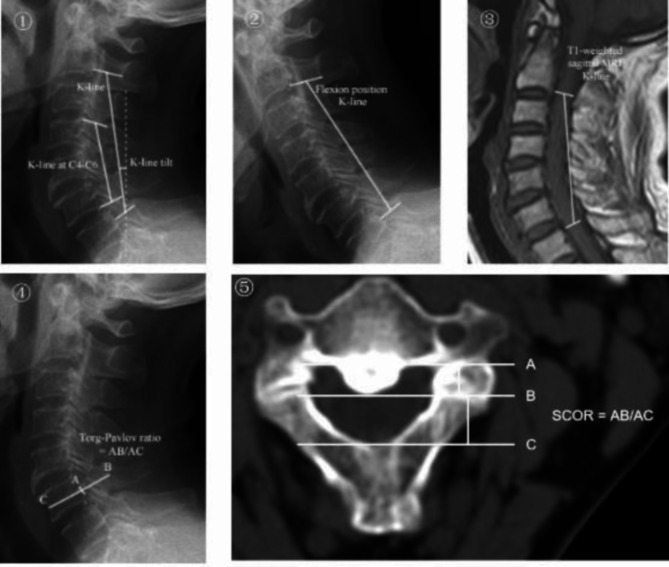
①. K-line is a straight line connecting the midpoints of the spinal canal at C2 and C7 on a neutral lateral cervical X-ray. K-line tilt refers to the angle between the K-line and a vertical line. K-line at C4-6 is a straight line connecting the midpoints of the spinal canal from C4 to C6. ②. K-line in the neck-flexed position is a straight line connecting the midpoints of the spinal canal at C2 and C7 on a flexion lateral cervical X-ray. ③. K-line on sagittal T1WI is defined as a line connecting the midpoints of the spinal cord at C2 and C7 on T1-weighted sagittal MRI. ④. Torg-Pavlov ratio is the ratio of the sagittal diameter of the spinal canal (AB) to the sagittal diameter of the vertebral body (AC). ⑤. SCOR is the ratio of the maximum thickness of COPLL (AB) to the anteroposterior diameter of the spinal canal (AC) at the same level
